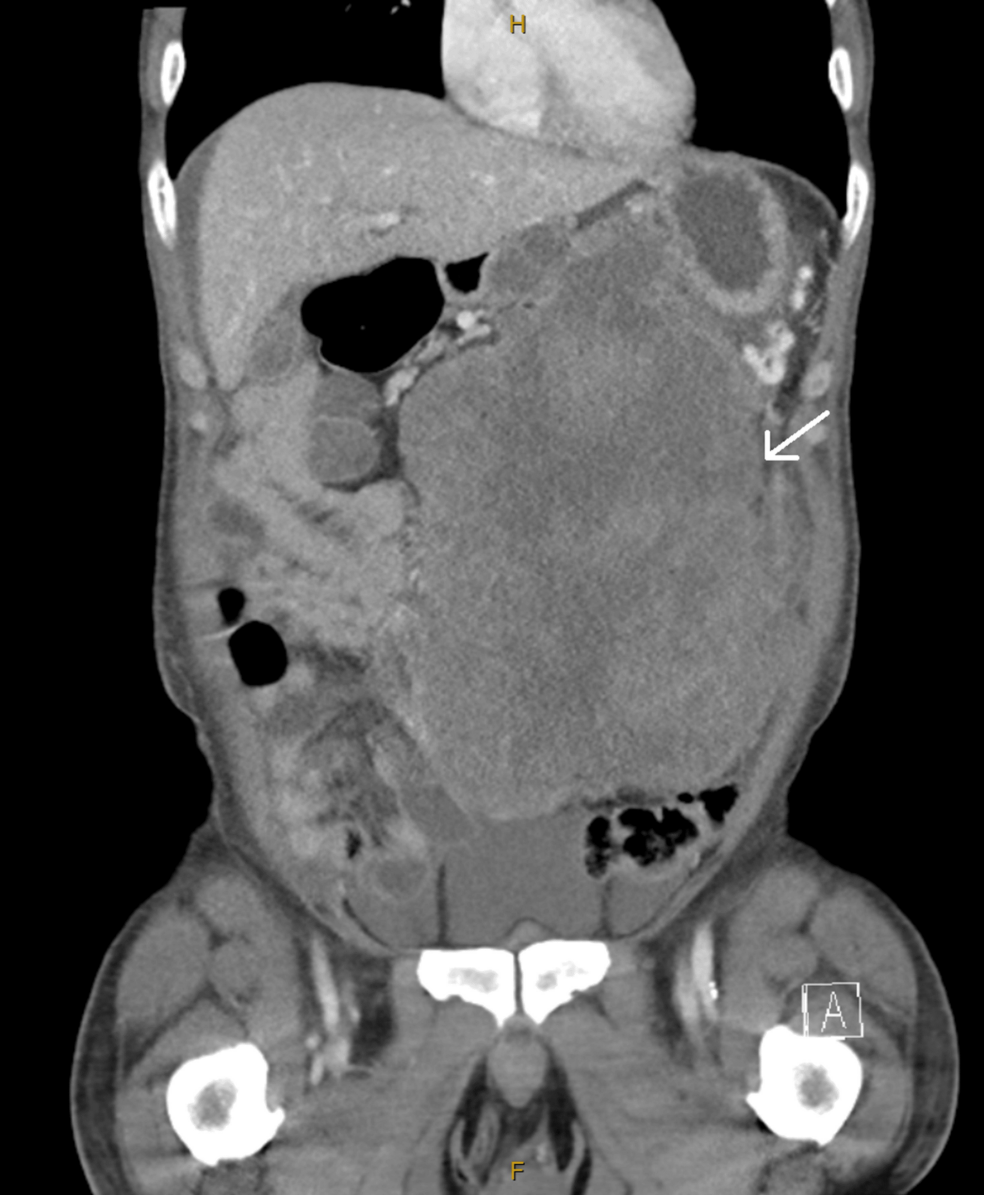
Coronal CT scan of the MRT White arrow indicating the large intra-abdominal tumor CT, computed tomography; MRT, malignant rhabdoid tumor
